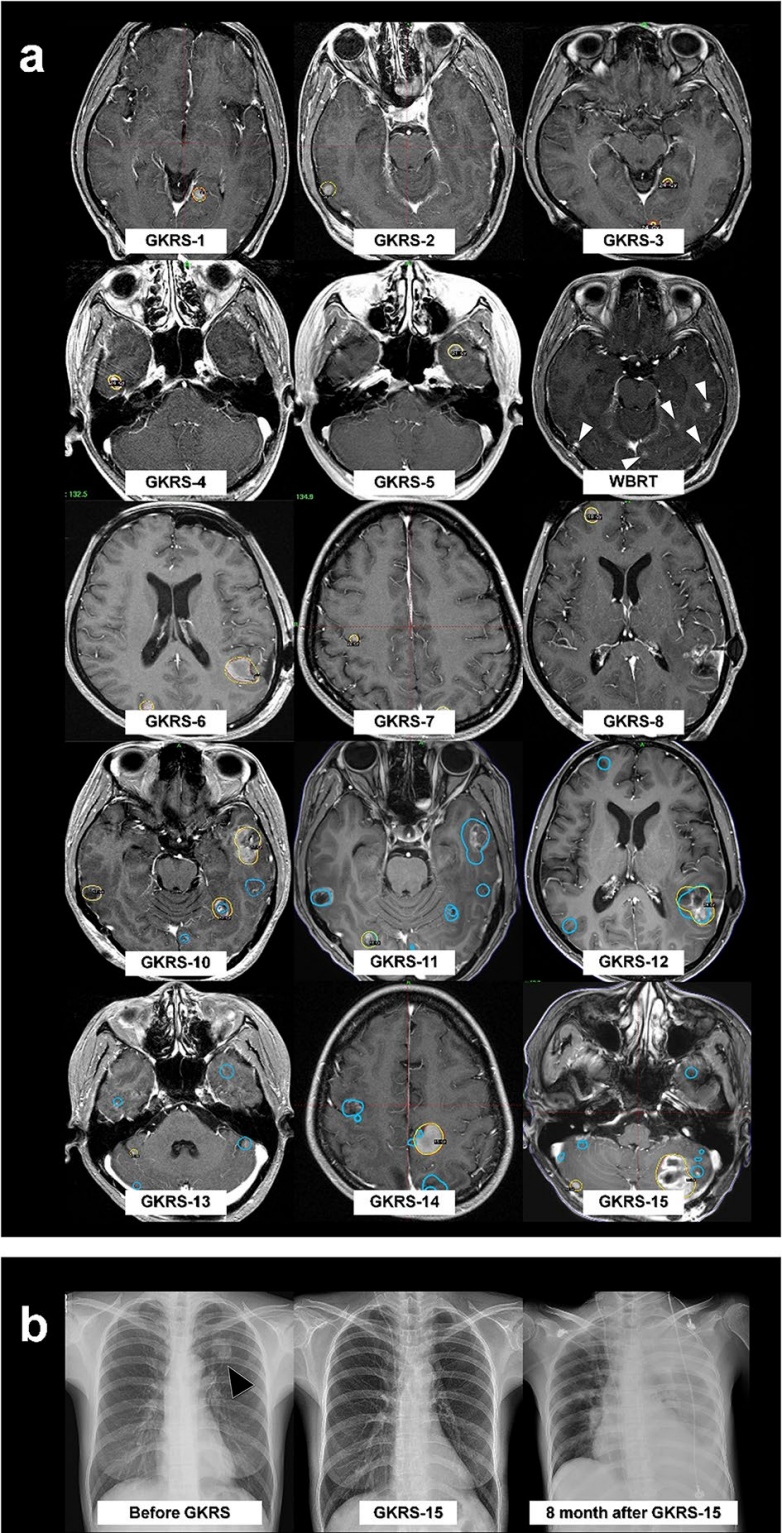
The clinical course of the a 36-year-old woman experiencing 15 GKRS procedures for BM from lung cancer. The patient was diagnosed with synchronous BM with adenocarcinoma of the lung. (a) Contrast-enhanced T1-weighted MR images for GKRS treatment. Target volume (red solid line) and prescription isodose volume (PIV) (yellow solid line) have appeared on each treatment planning image. PIVs of previously irradiated BM by gamma knife is marked with blue solid lines. The numerous lesions are indicated with white arrowheads resulting in an additional WBRT after the fifth GKRS. An Ommaya reservoir is inserted into a large cystic BM at the sixth GKRS. The newly developed hemorrhagic BM is shown in the cerebellum of the patient, resulting in metastasectomy and ventriculo-peritoneal shunt surgery after the 14th GKRS. (b) The chest X-rays showing lung cancer (black arrowhead) without systemic progression until the 15th GKRS (114 months after the first GKRS). Chest X-ray showing a large amount of pulmonary effusion on the left side after her death, following acute respiratory failure due to septic shock subsequent to 8 months of the last GKRS. *Data from the 9th GKRS session are lost.
